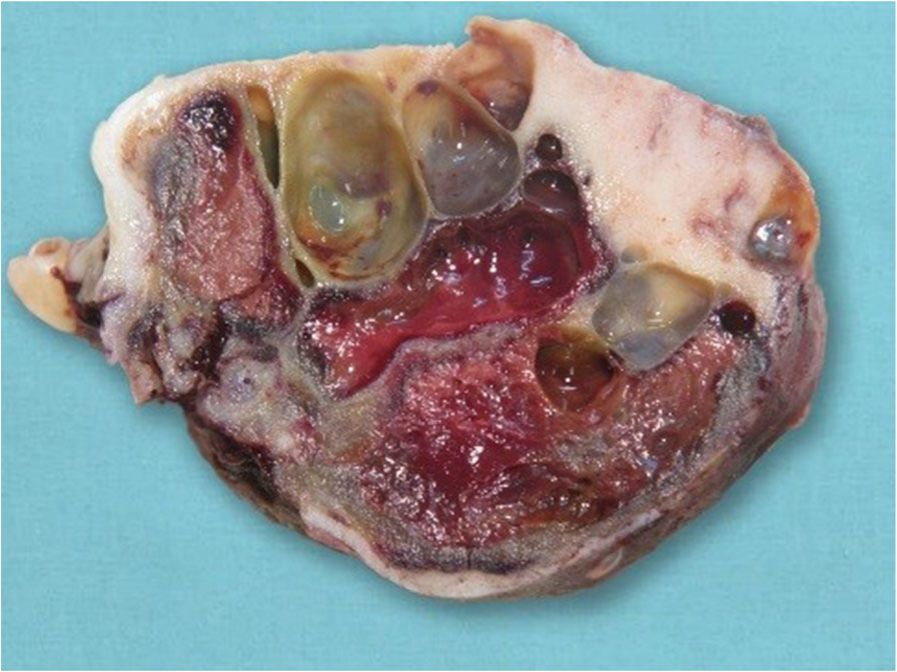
Sagittal cross-section of the tumour reveals many cystic spaces in the solid part, mirroring the computed tomography findings
